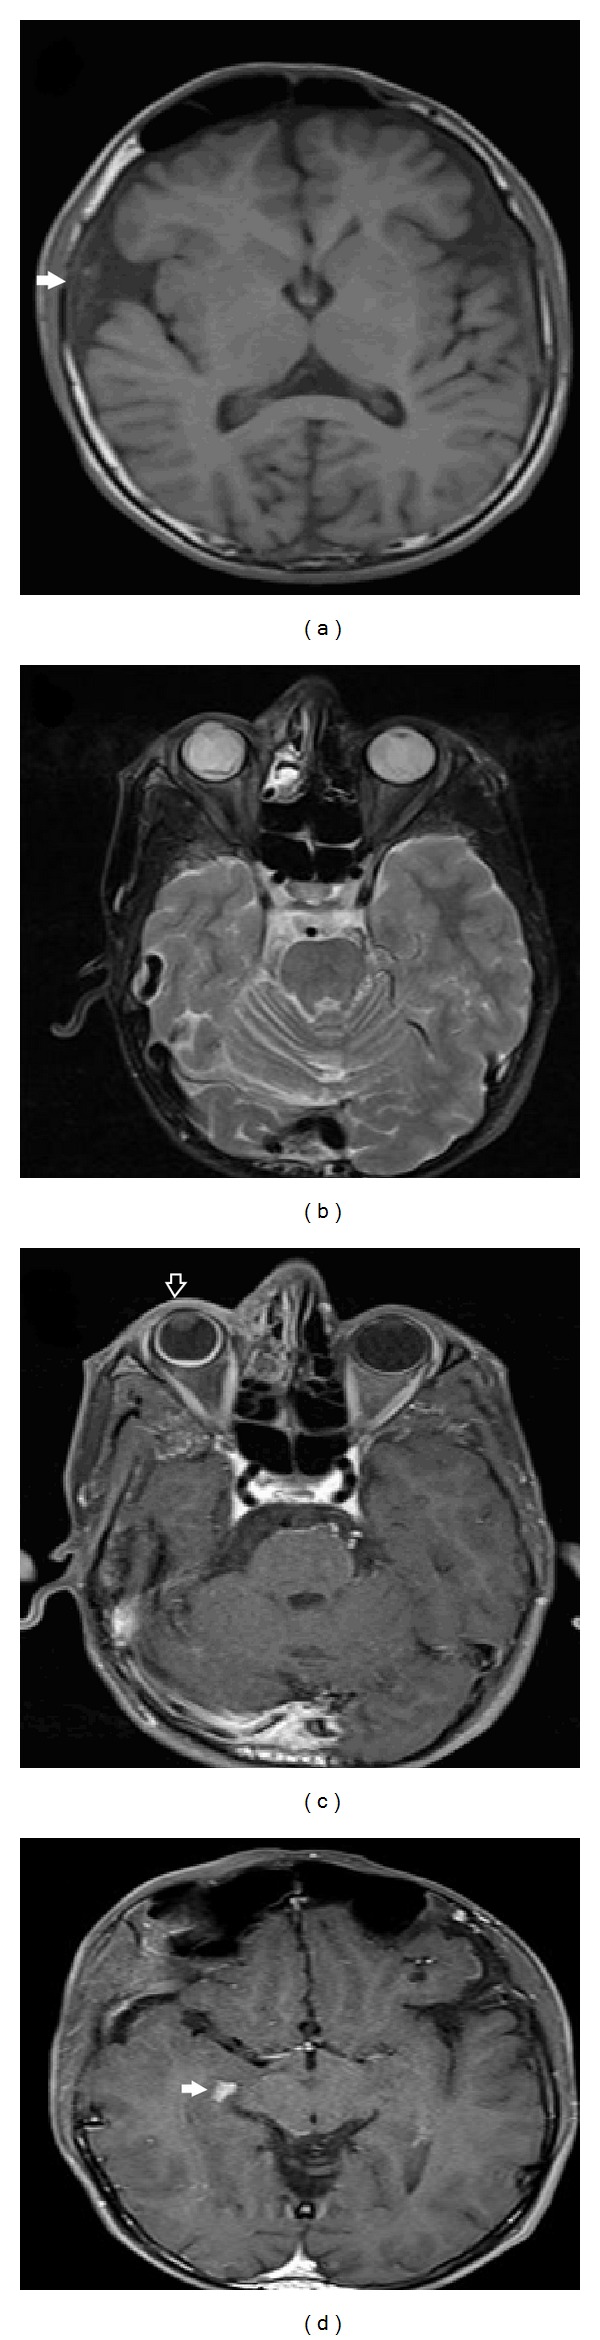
Cranial magnetic resonance imaging studies showed cortical atrophy discordant to his age (a), asymmetric vascular dilatations in the right hemisphere (b), hypertrophy in the right periorbital soft tissue (c), and choroidal plexus (d).
